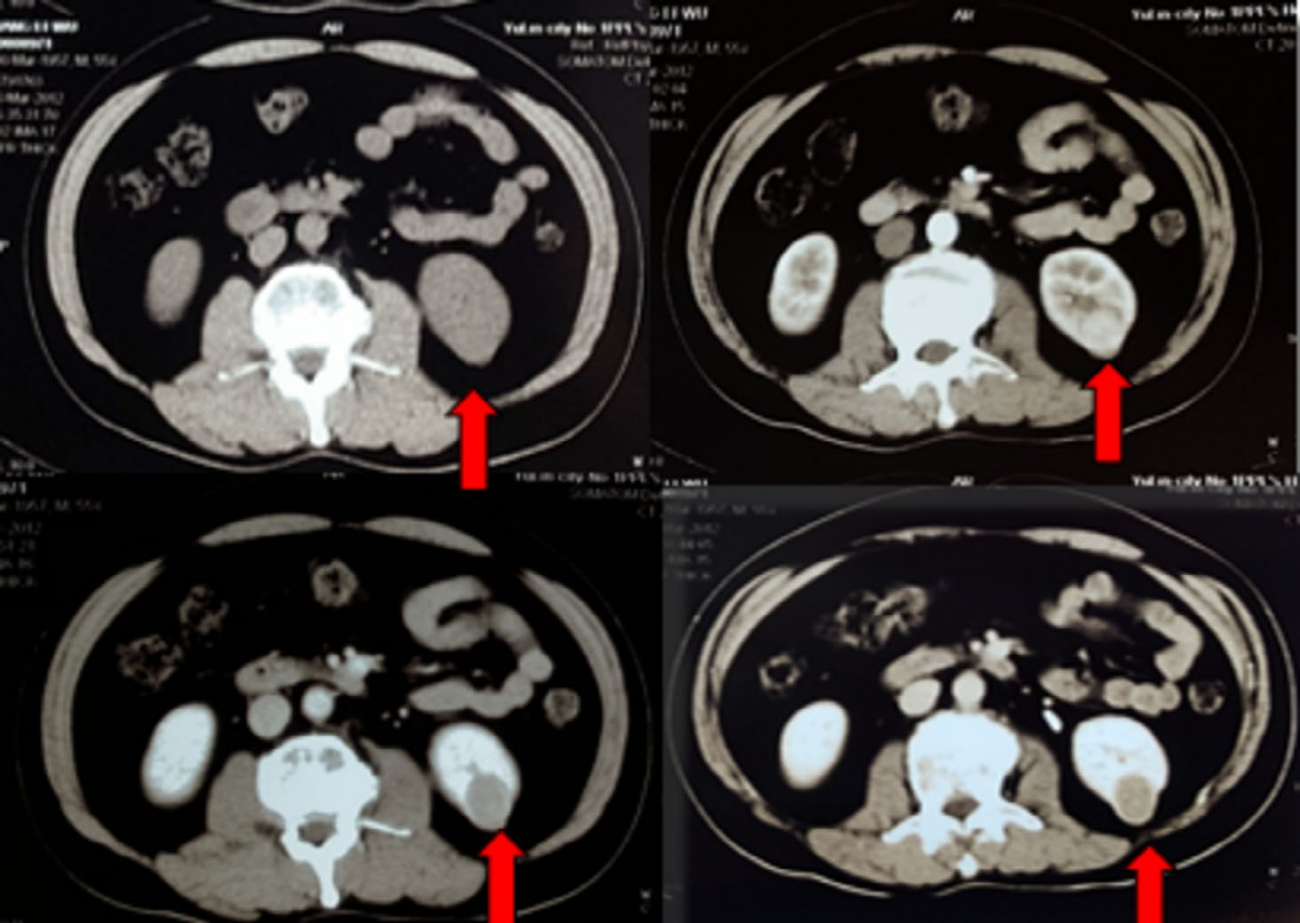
Urinary CT examination on first admission.
